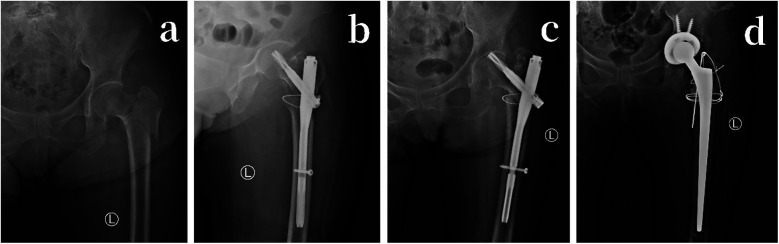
(a) 73-year-old female with a fall-induced intertrochanteric fracture of the left femur. (a) Preoperative anteroposterior x-ray of the left hip joint. (b) Postoperative first-day anteroposterior x-ray of the left hip joint, using PFNA internal fixation with external lateral wall bundling with titanium cables. (c) Postoperative 5-month anteroposterior x-ray of the left hip joint, showing proximal screw cutting of PFNA. (d) Removal of PFNA internal fixation, followed by total hip joint replacement surgery; postoperative first-day anteroposterior x-ray of the left hip joint.
